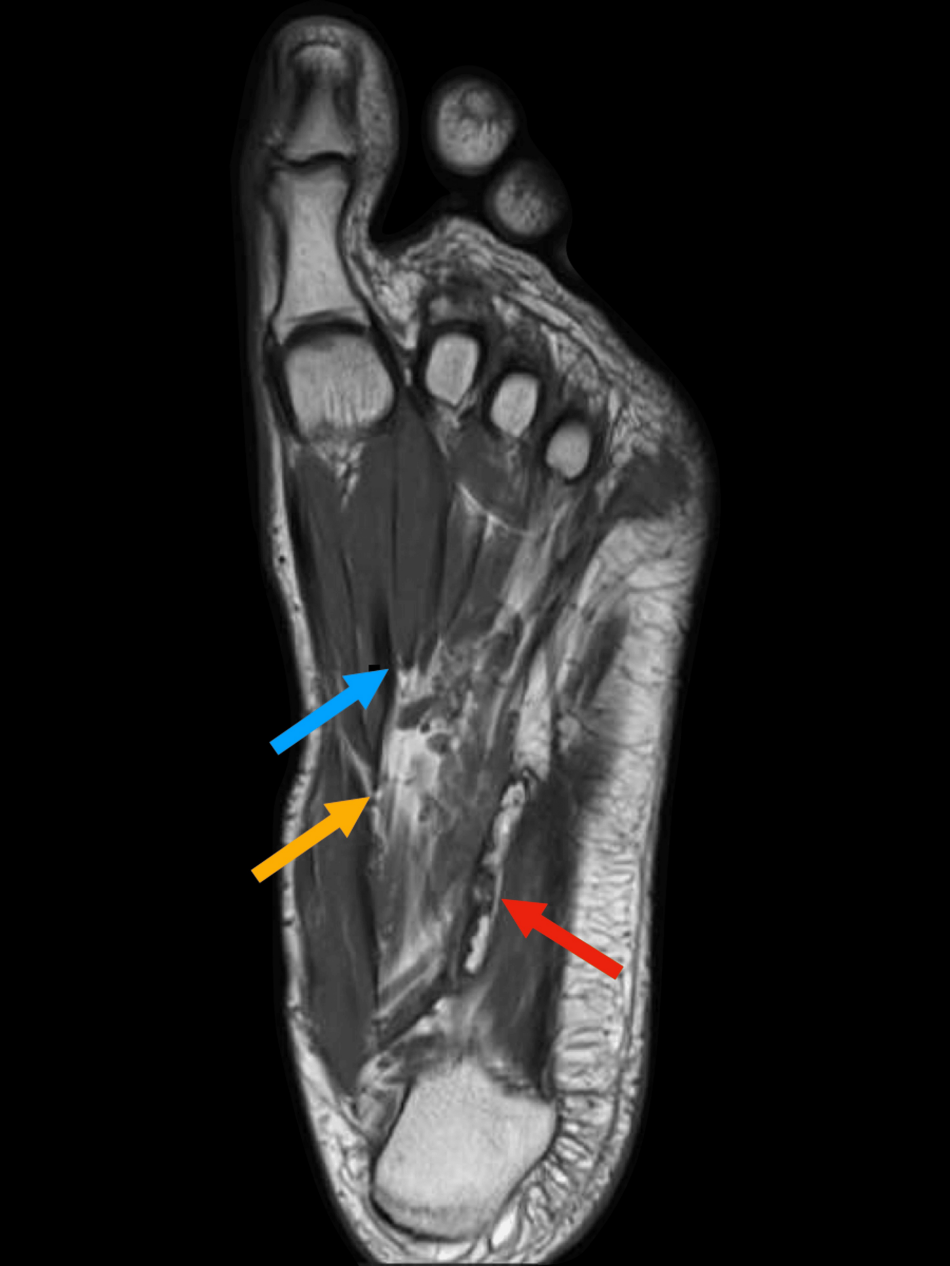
T1-weighted image of the left foot. Intermediate signal of the pathological abnormality measuring 6.9 x 2.9 cm (yellow arrow), with invasion of the abductor digiti minimi tendon (red arrow) and involvement of the adductor hallucis muscle (blue arrow).
